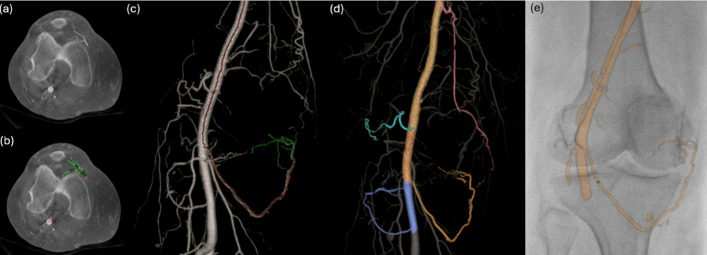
Advanced planning on CBCT using Virtual Injection technology (Embo ASSIST AI, GE HealthCare) a Contrasted CBCT with a 10-mm MIP axial view demonstrates initial opacification of the genicular branches from a superficial femoral artery injection. b Virtual Injection planning. A single-click selection of the injection point automatically highlights the target artery feeder (in green) and the catheter path (in red). c Zero-click automatic vessel segmentation generates a 3D vascular model displaying the full arterial tree, including the highlighting target artery feeder (in green) and catheter path (in red). d Segmented model with selected genicular arteries, including the descending genicular artery (red), superior lateral artery (cyan), inferior lateral (blue), and inferior medial artery (orange). e Automatic 3D model overlay onto live fluoroscopy provides augmented guidance for catheter navigation and embolization
